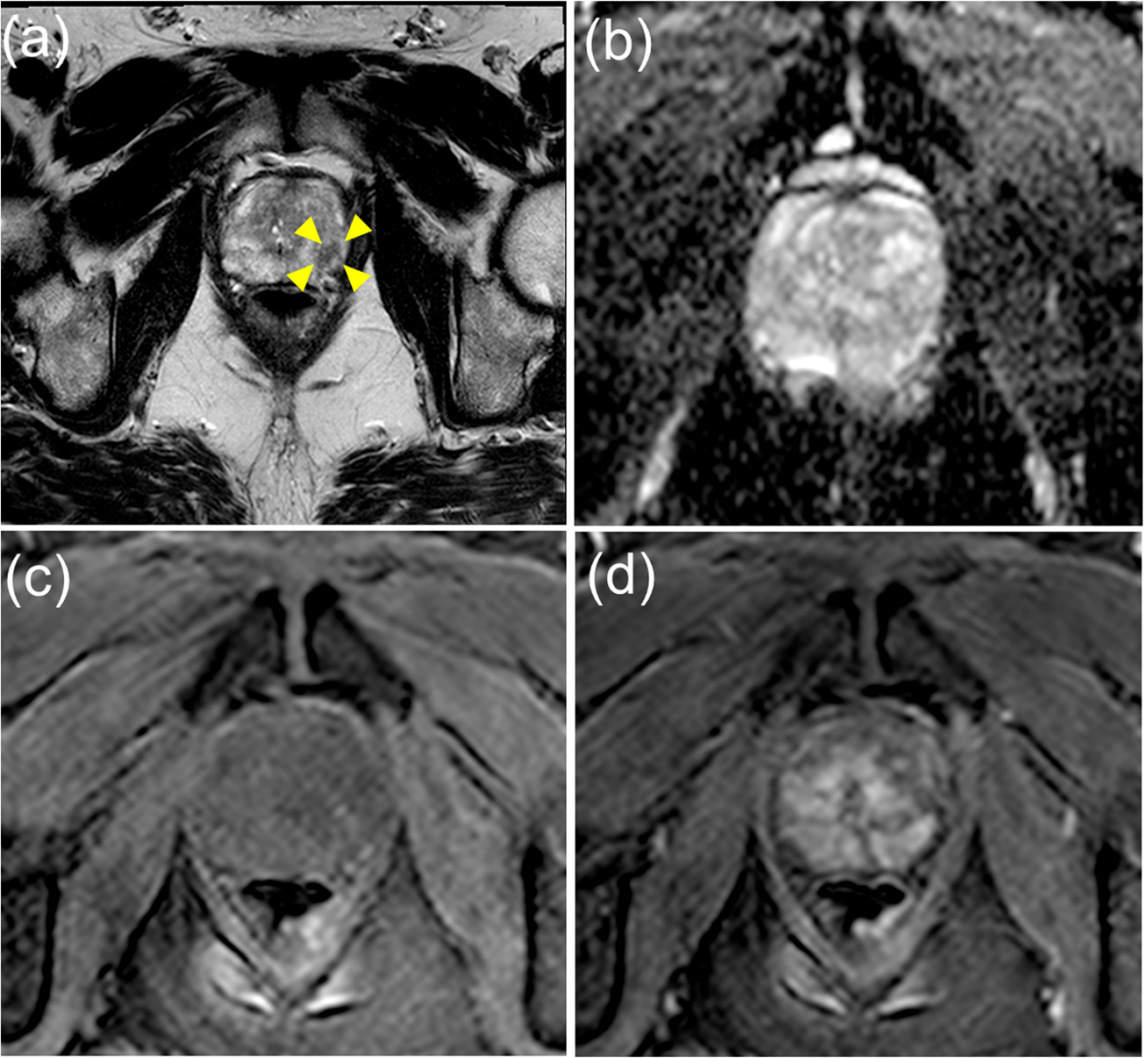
Axial multi-parametric MR images (a T2-weighted, b apparent diffusion coefficient map, c pre-contrast T1 and d early post-contrast T1) of a region in the peripheral zone scored as equivocal (diffused lesion; positive tumour TPM biopsy) by the radiologists
